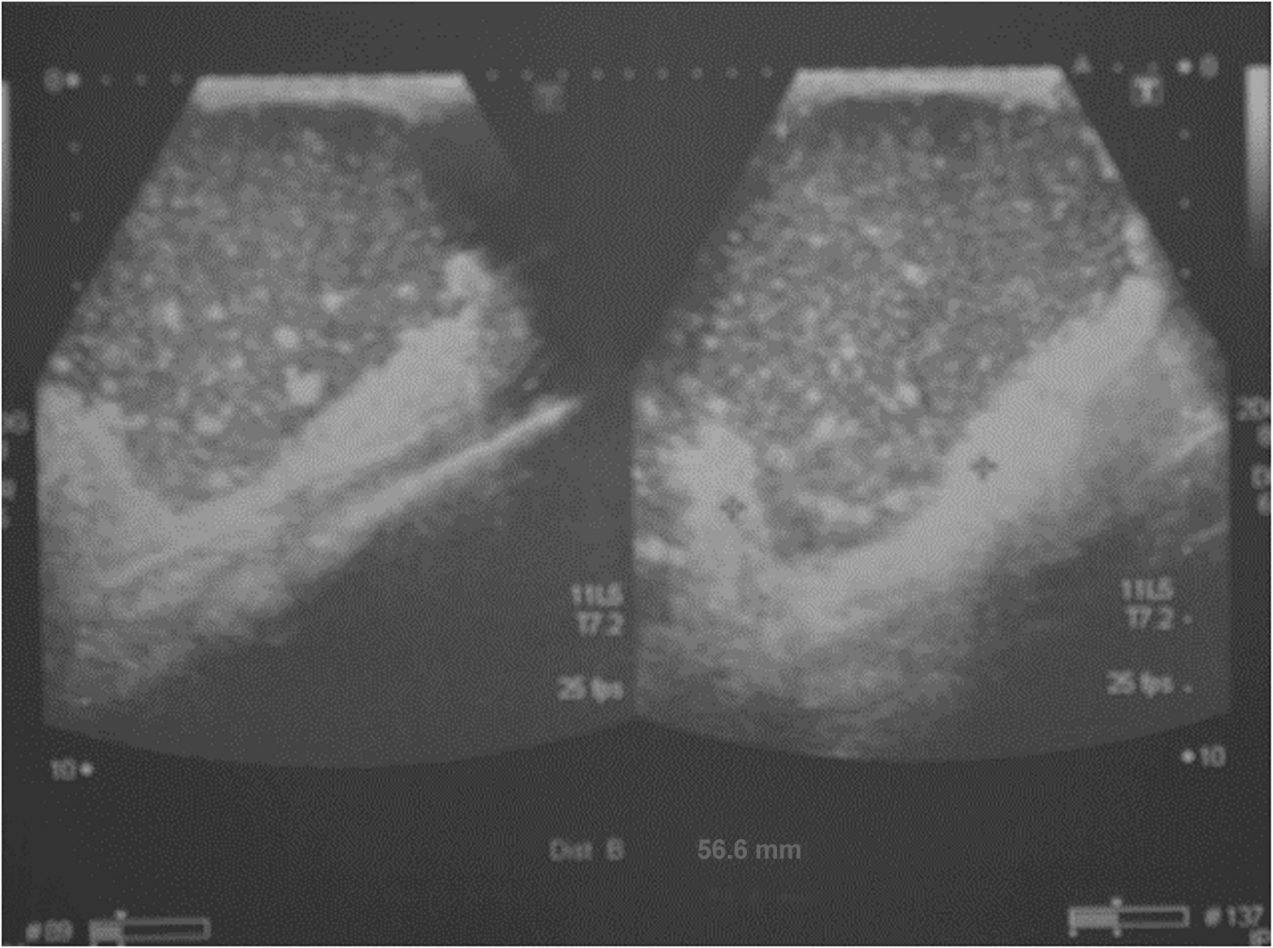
Chest CT scan showing numerous multiple lung nodules with multiple dot-in sign characteristic for mycetoma.
